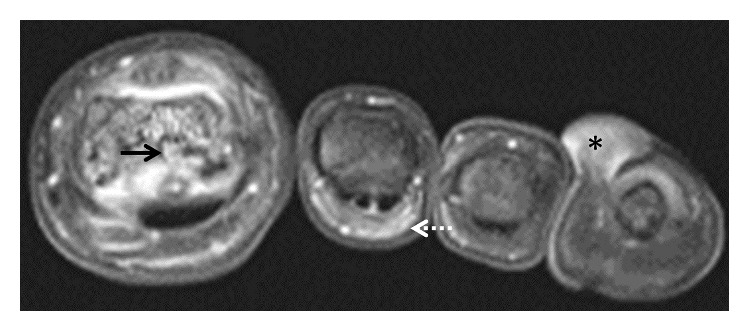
Coronal T1 fat-saturated magnetic resonance imaging (MRI) of the foot obtained with gadolinium contrast administration demonstrated marginal erosions at the great toe interphalangeal joint, associated synovitis, and bone marrow enhancement (black arrows). Enhancing inflammatory changes in the second and fourth toe plantar soft tissues were present (dashed white arrows). One of the several keloids is seen along the medial margin of the fourth toe at the level of the nail with moderate heterogeneous enhancement (asterisk).
